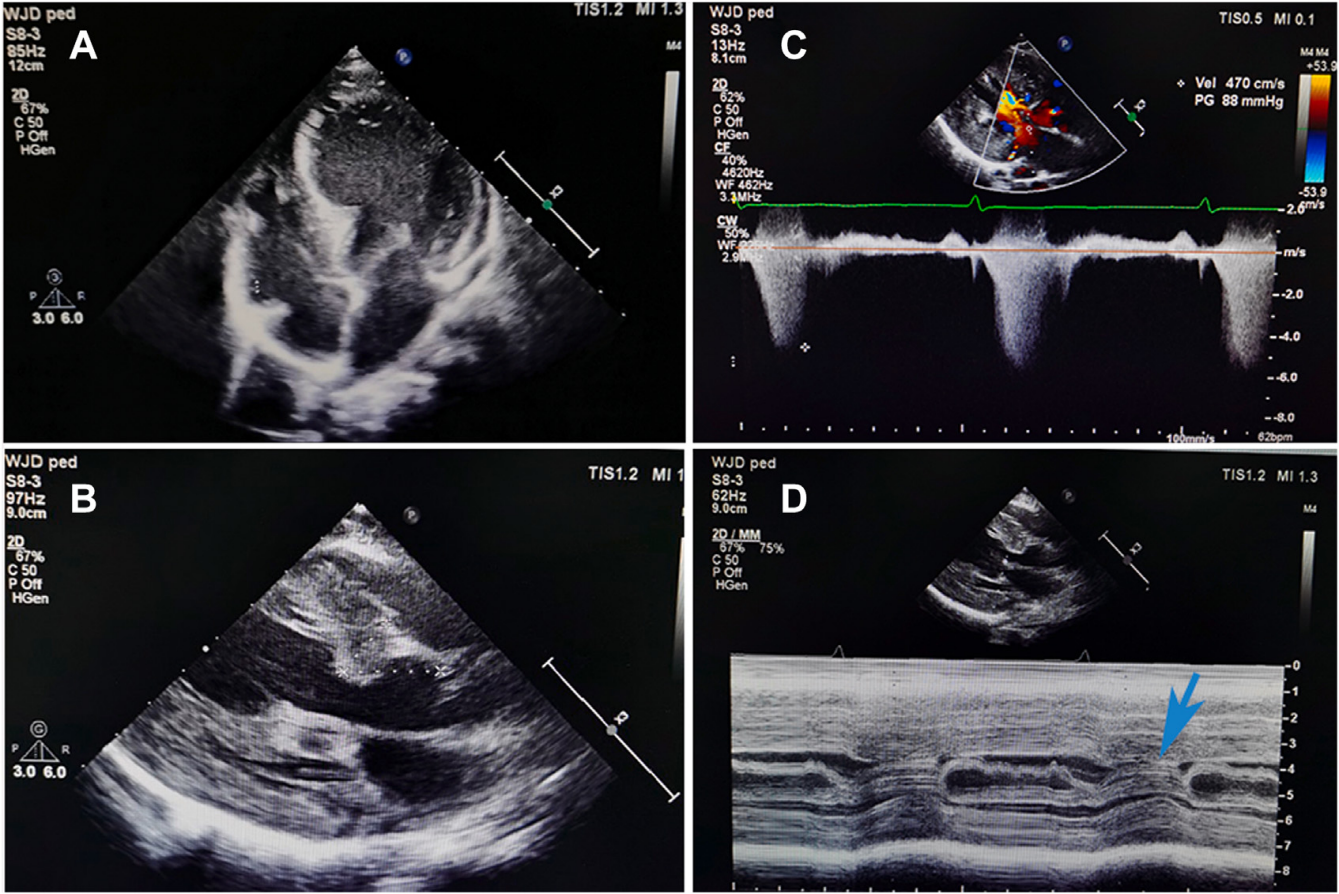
Transthoracic Echocardiogram (A) Four-chamber view at end diastole and (B) parasternal long-axis view at end systole show anatomical findings of increased wall thickness. (C) Continuous-wave Doppler (C) reveals a maximum velocity of 4.7 m/s and a calculated peak gradient of 88 mm Hg through the left ventricular outflow tract (LVOT) at rest. (D) M-mode echocardiogram shows systolic anterior motion of the mitral valve (blue arrow), indicating LVOT obstruction.
